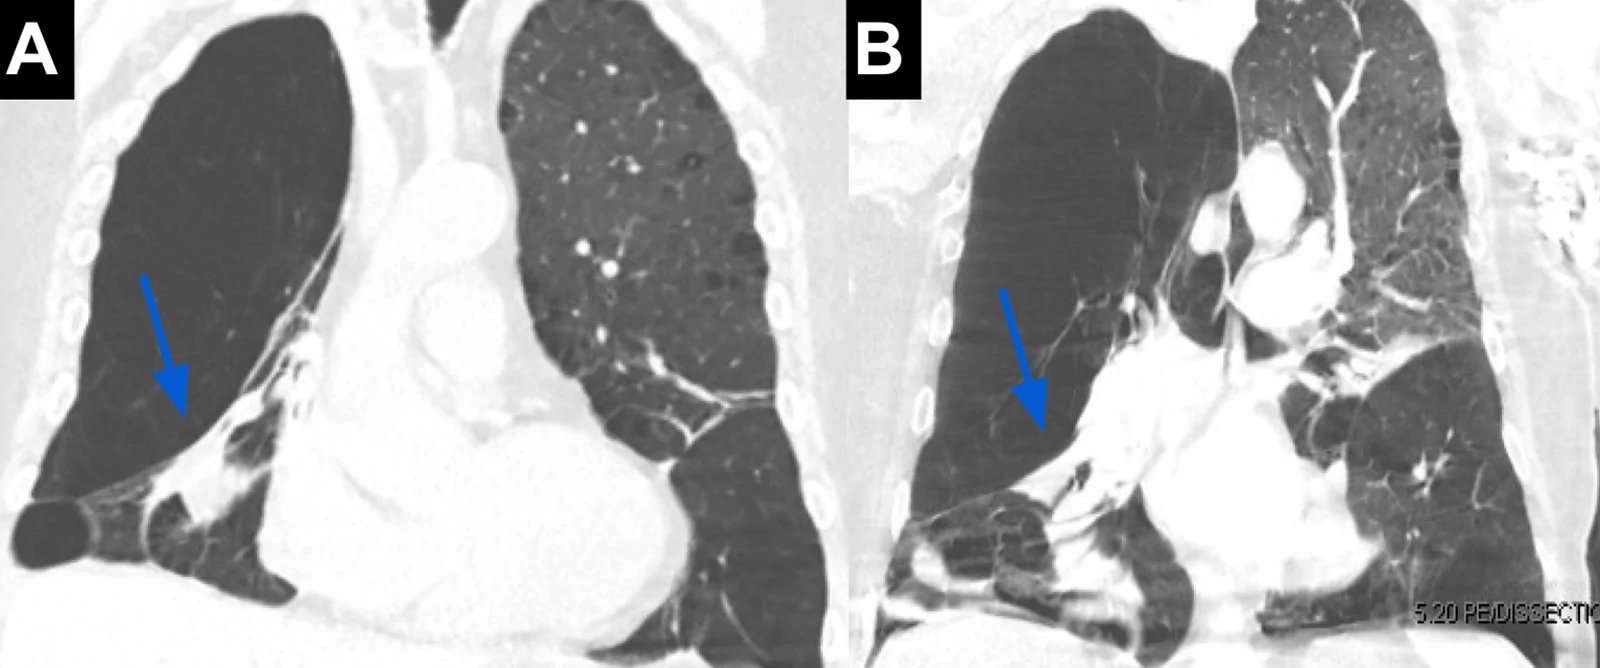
Coronal chest imaging demonstrating concern for torsion. (A) CT chest with intravenous contrast. The blue arrow indicates compressed and medially displaced right lower lobe parenchyma along the medial margin of the large bulla, which extends into the right upper lung region. (B) CT angiography. The blue arrow shows the abnormal orientation and swirling of the right hilar vessels.
